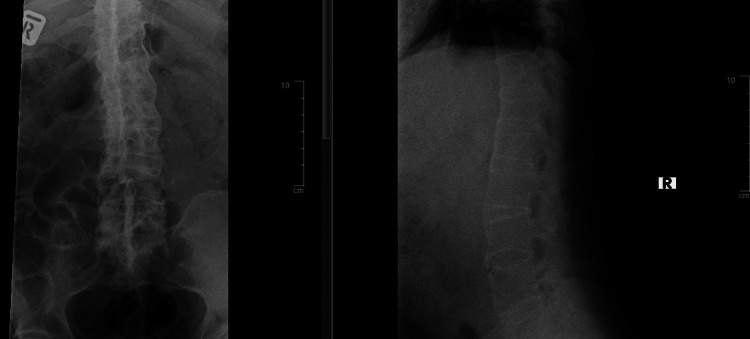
Plain lumbosacral radiograph: no visible fracture with "bamboo spine"
